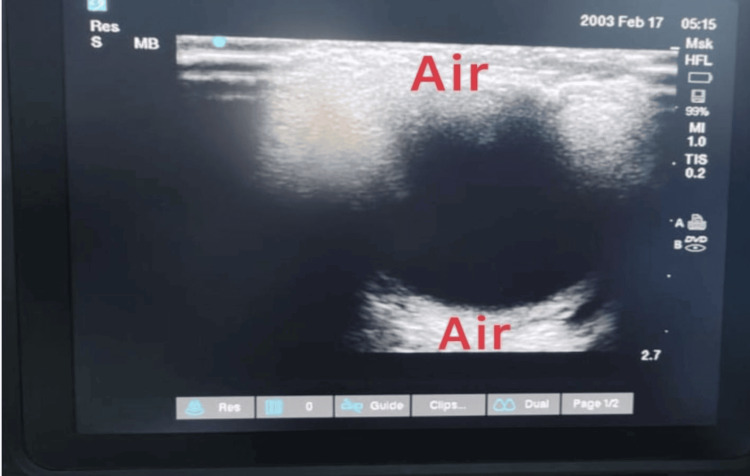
Point-of-care ultrasound (POCUS) showing air around the eyeball confirming the diagnosis of periorbital emphysema
